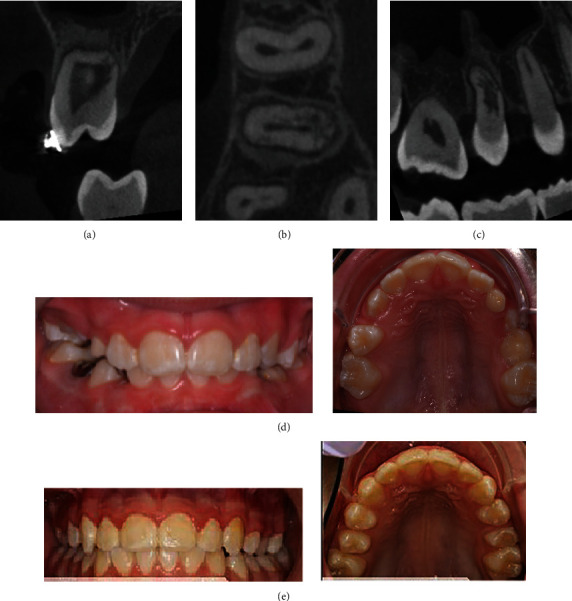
Follow-up CBCT eight months after surgical exposure. Tooth #4 eruption near to occlusal plane: (a) coronal, (b) axial, and (c) sagittal. Significant progression of resorption. (d) Initial intraoral images and (e) final intraoral image.
